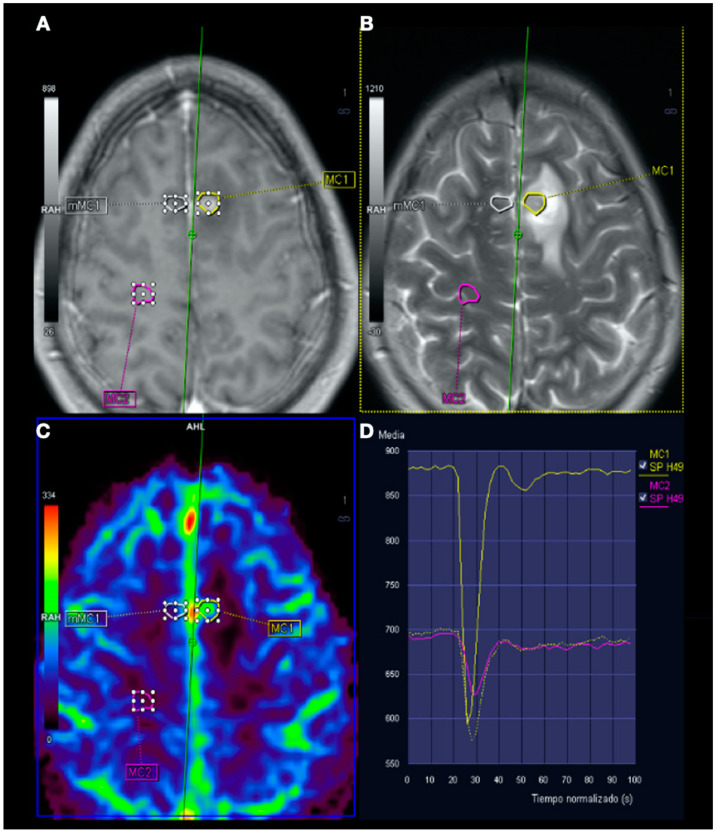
Magnetic resonance imaging (MRI) perfusion: Image (A), Gd T1WI; image (B), T2WI; image (C), MRI perfusion map corresponding to the corrected cerebral blood volume (CCBV); image (D), average curve. In the contrast enhancement area, there is an increase in cerebral blood volume (CBV) in relation to the contralateral side and normal subcortical white matter. In the average curves, the area under the curve is higher in the enhancement zone than in the other two zones.
